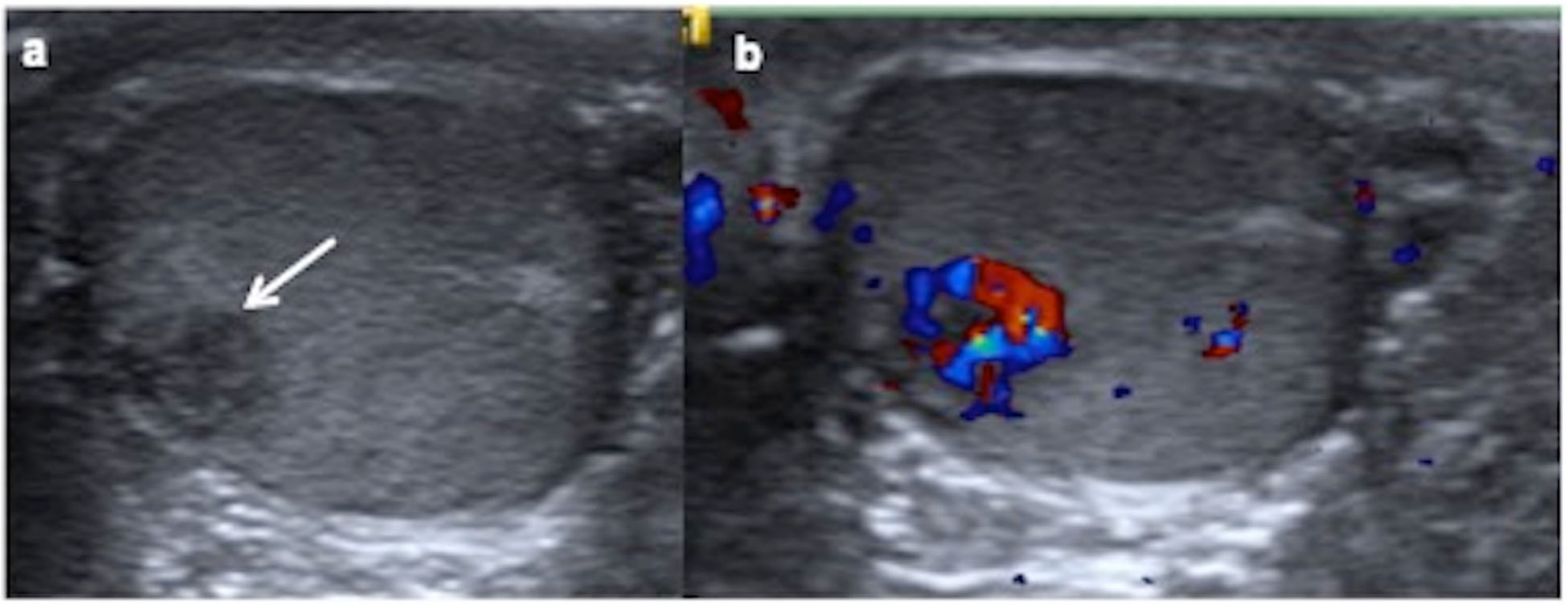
Leydig cell tumour. A 7-year-old boy with precious puberty. High level of testosterone (3.762 ng/mL). a Transverse ultrasound view of the right testis shows a hypoechoic lesion (arrow). b Colour Doppler imaging demonstrates an increased tumour vascularization. A testis sparing surgery with tumour enucleation is practised. Leydig cell tumour is the definitive pathologic diagnosis
